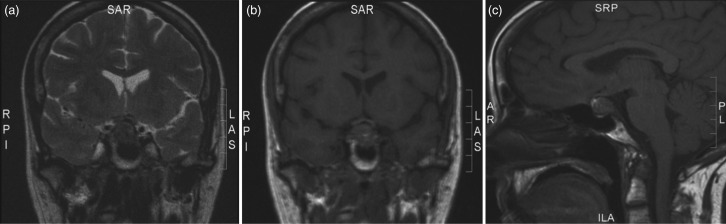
2006 MRI pituitary: (a) coronal T2WI, (b) coronal T1WI and (c) sagittal T1WI respectively.
